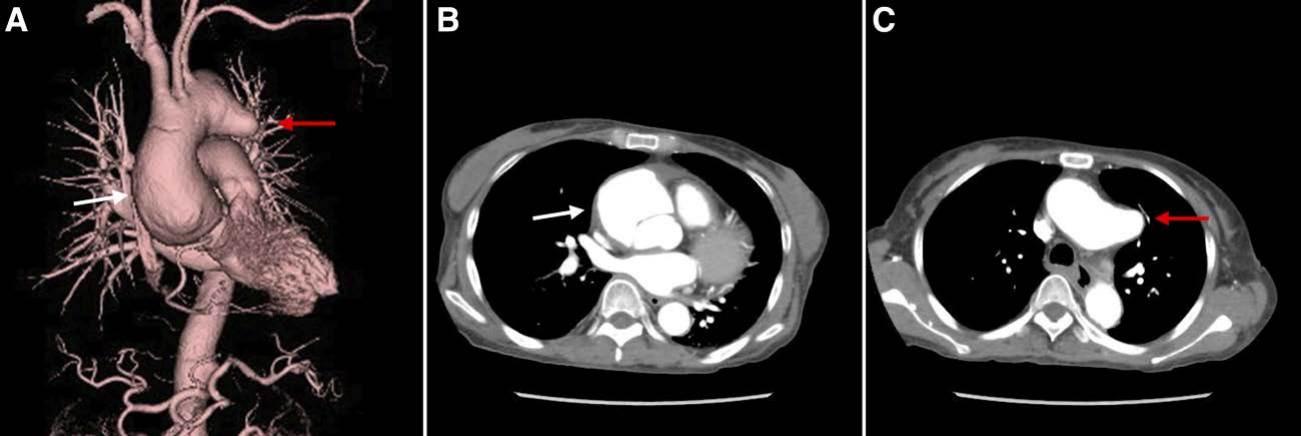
Acute aortic dissection (DeBakey type II) and true saccular aneurysm. The patient was a 55-year-old woman with a 33-year history of SLE. She was taking 15 mg of prednisolone daily. She also had an IVC filter implanted for deep vein thrombosis. 3D-CT showed aortic dissection (DeBakey type II, white arrow) and a true saccular aneurysm at the distal arch (red arrow). 3D-CT = three-dimensional computed tomography, IVC = inferior vena cava, SLE = systemic lupus erythematosus.
